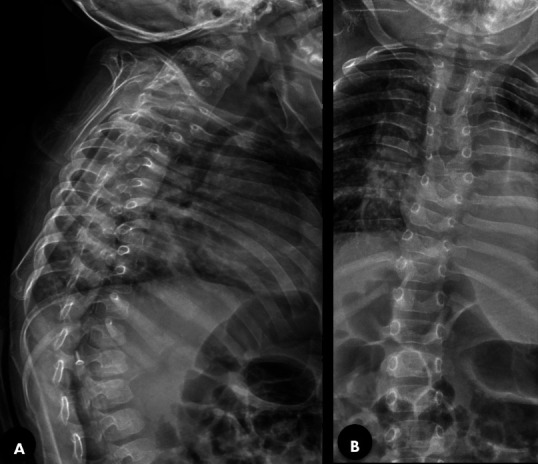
A) Cervicothoracic spine radiograph showing C3-C4 fusion, B) Thoracolumbar radiograph showing scoliosis.
